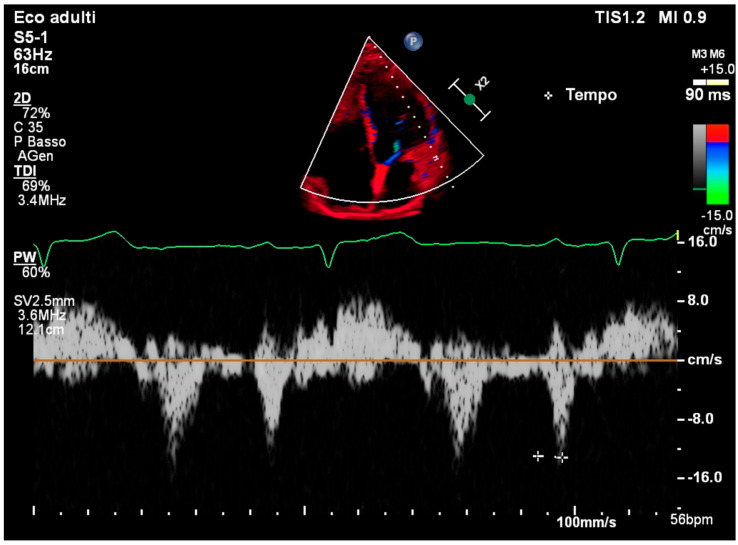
Tissue Doppler imaging of the lateral mitral annulus showing normal PA—TDI interval.
